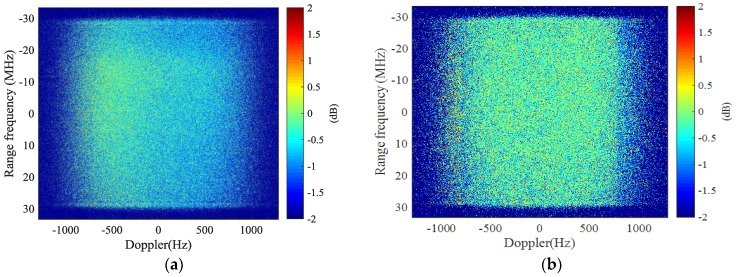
Amplitude imbalances in the 2-D spectral domain before and after imbalance correction (calibration bandwidths: 60 MHz in range and 1.58 kHz in Doppler): (a) before imbalance correction; and (b) after imbalance correction.
